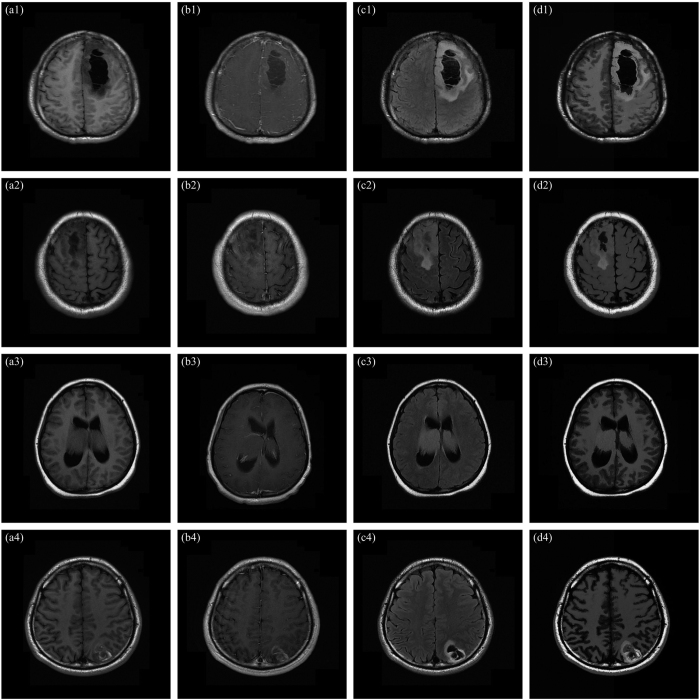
Original primary tumor images and improved results obtained by using our method. (a1–a4), (b1–b4), (c1–c4), and (d1–d4) Original T1-weighted, contrast-enhanced T1-weighted, T2-FLAIR images, and improved results with the AIFE method.
